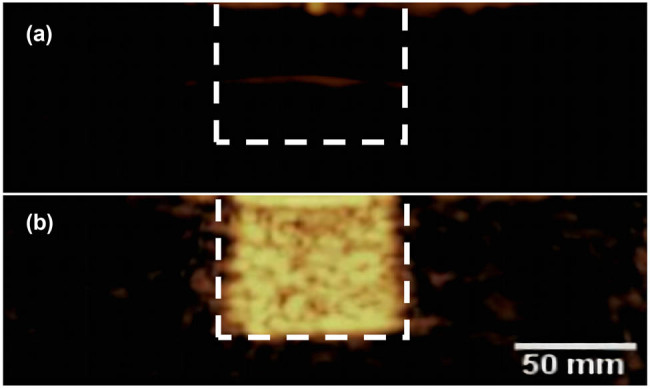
(a) Contrast-enhanced ultrasound image of PBS buffer shows an anechoic dark area. (b) DOX-NB contrast-enhanced ultrasound image shows a dense and uniform high enhanced area.
